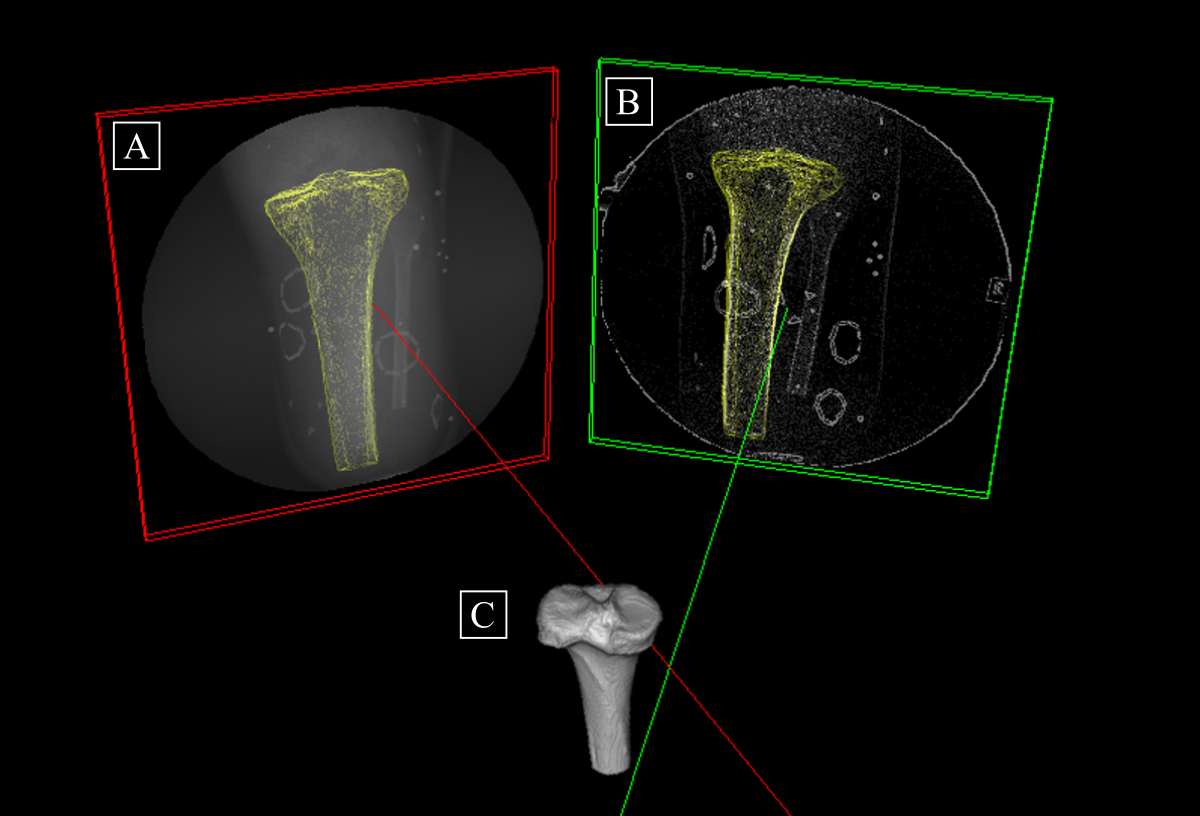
A 3D representation of the synchronized x-rays. The inline x-ray image is the red frame (A), whereas the offset x-ray image is the green frame (B). The 3D residual tibia (C) is reconstructed from computed tomography (CT) data. The red and green lines are the perpendiculars from the x-ray image planes to the x-ray sources. The outlines of the tibia (shown in yellow) superimposed over each x-ray image are created by casting rays from each x-ray source through the CT tibia to the x-ray planes.
